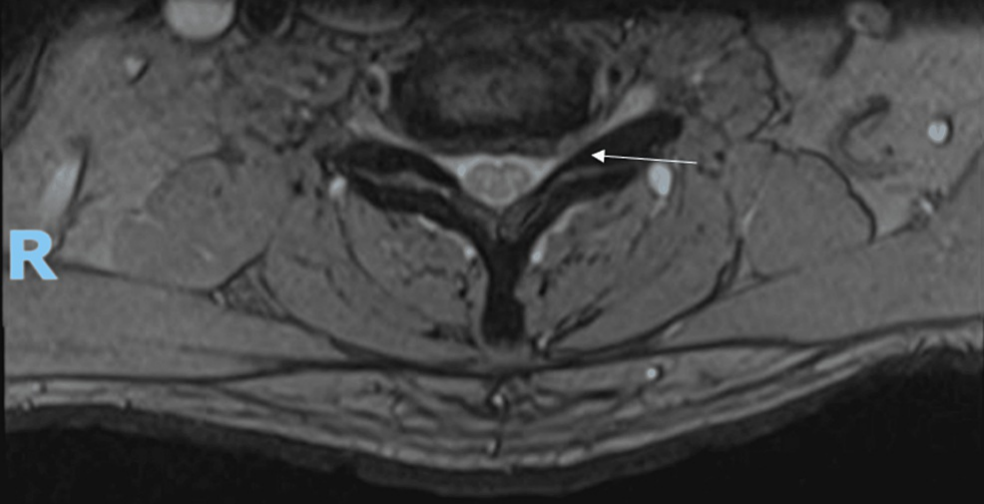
Preoperative T2-weighted MRI of the cervical spine (axial view) Severely narrowed left C6/C7 neural exit canal (arrow) MRI: magnetic resonance imaging
